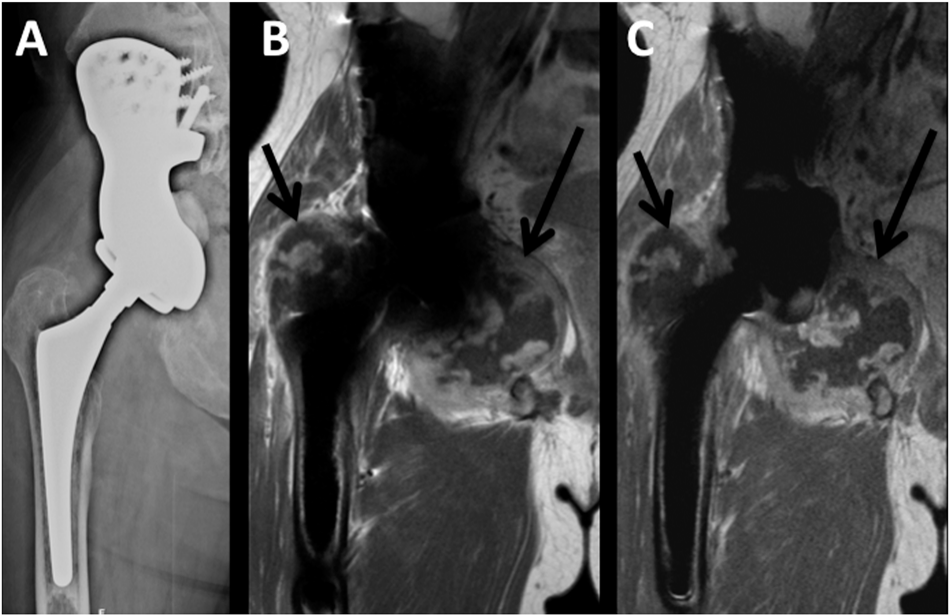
Tumor recurrence. Hip endoprosthesis with tumor recurrence of a chondrosarcoma in a 45 year old male patient. (A) a.p. radiograph; (B) Coronal contrast enhanced T1-w image with conventional artifact reducing MR techniques; (C) Coronal contrast enhanced T1-w image with VAT&SEMAC techniques.
